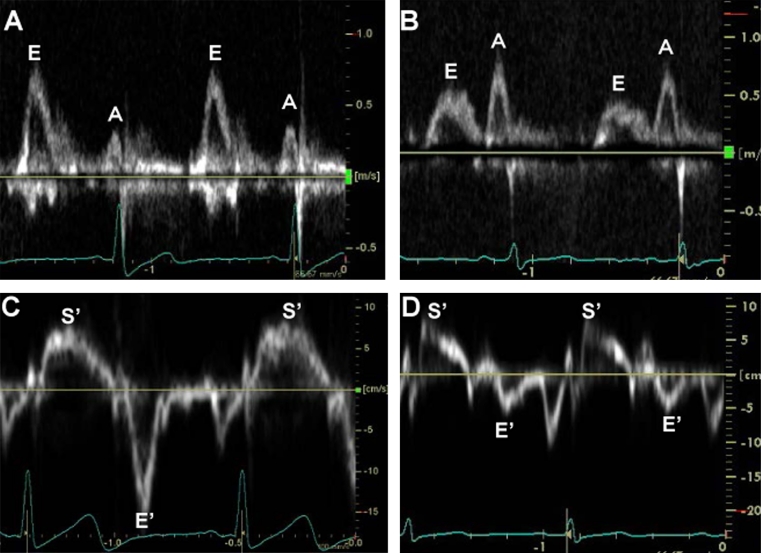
Examples of Doppler echocardiography in a healthy subject and a T2DM patient. Transmitral flow patterns are shown for a healthy subject (a) and a T2DM patient (b). Peak velocities during early diastole (*E*) and late diastole (*A*) are shown. *E*/*A* ratios are 2.2 and 0.6 in a, b, respectively. c, d show tissue Doppler imaging, with positioning of sample volume at the septal mitral annulus, in a healthy subject and a T2DM patient, respectively. The diabetic patient (d) had lower peak velocities during systole (*S*′) and early diastole (*E*′) (7.5 and 6.0 cm/s, respectively) than those in the healthy subject (c 8.5 and 15.0 cm/s, respectively)
